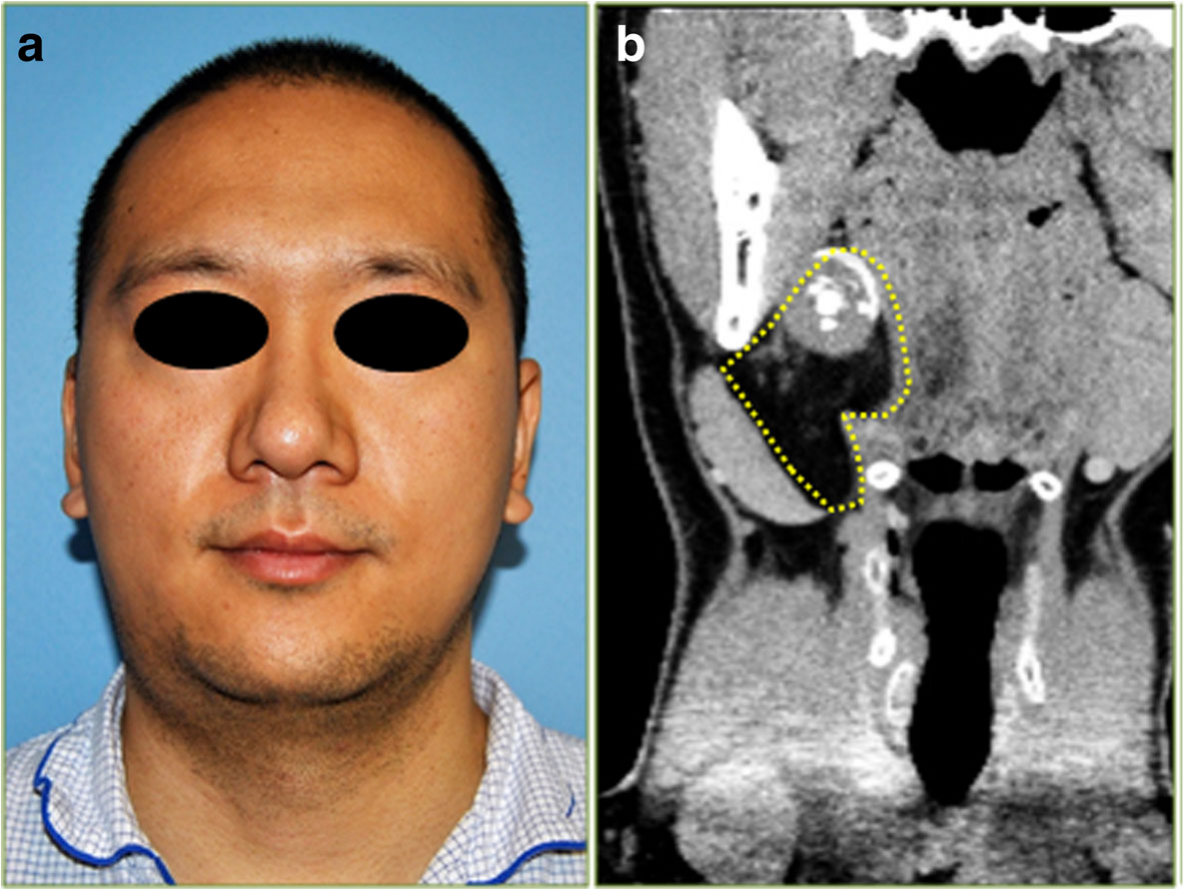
Extraoral photograph shows a mass in the patient’s right submandibular region (a). Coronal head CT scan shows a mixed lesion (outlined by the dotted line) near the right submandibular gland (b)
